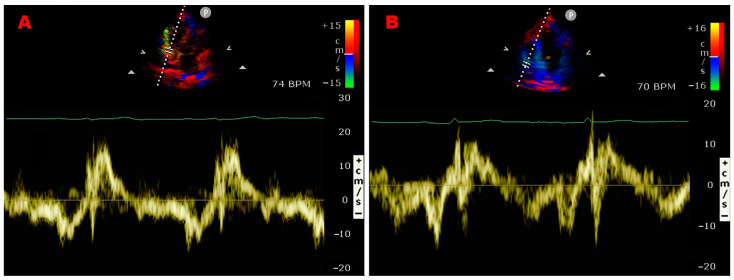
Examples of PW-TDI assessment of the systolic infolding of the lipomatous right atrioventricular junction (A) and of the RA infero-lateral wall (B). Spectral PW-TDI allows for the detection of a pattern of concordant motion in synchrony with the phases of the cardiac cycle, thus indicating RA pseudomasses. PW, pulsed wave; RA, right atrial; TDI, tissue Doppler imaging.
